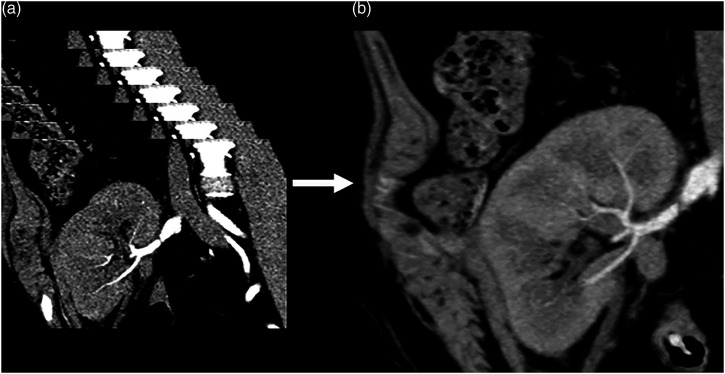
(a, b). Low resolution CT images reconstructed from several time-points (a). By merging data from several time-points, a time-resolved merged data set with high image quality can be reconstructed (b).
